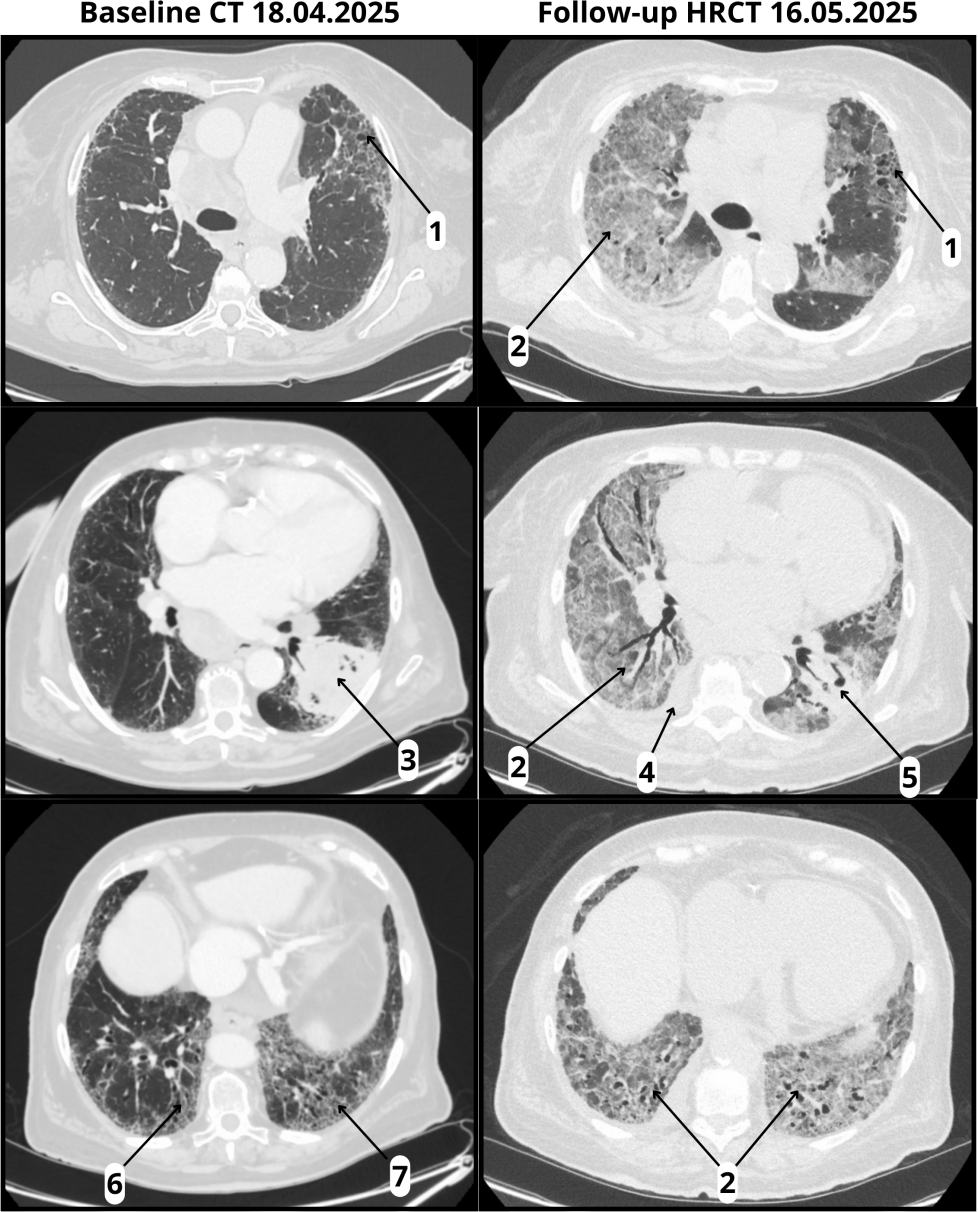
Baseline CT and follow-up HRCT. Baseline CT (18 April 2025) and follow-up HRCT (16 May 2025, performed 22 days after pembrolizumab infusion): (1) Honeycombing; (2) ground-glass opacities (absent at baseline, new at follow-up); (3) tumor in the left lower lobe with pleural involvement; (4) right pleural effusion (new at follow-up); (5) reduction in tumor size (follow-up); (6) traction bronchiectasis; (7) volume loss.
